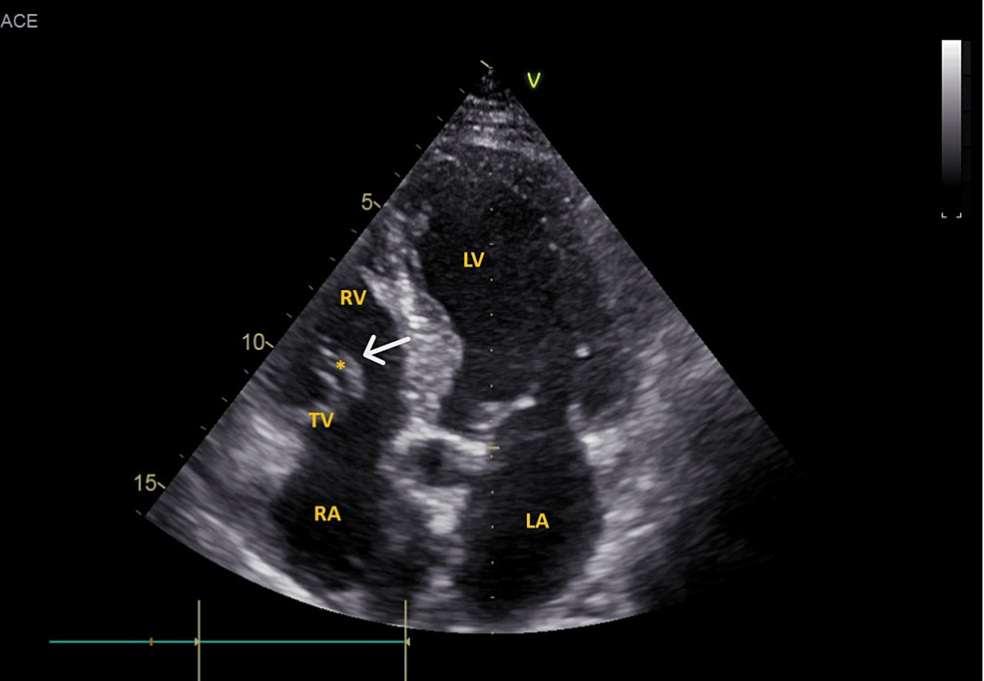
TTE showing a mobile mass on the tricuspid valve RV: right ventricle; LV: left ventricle; LA: left atrium; RA: right atrium; TV: tricuspid valve; *: added structure/vegetation; TTE: transthoracic echocardiogram
